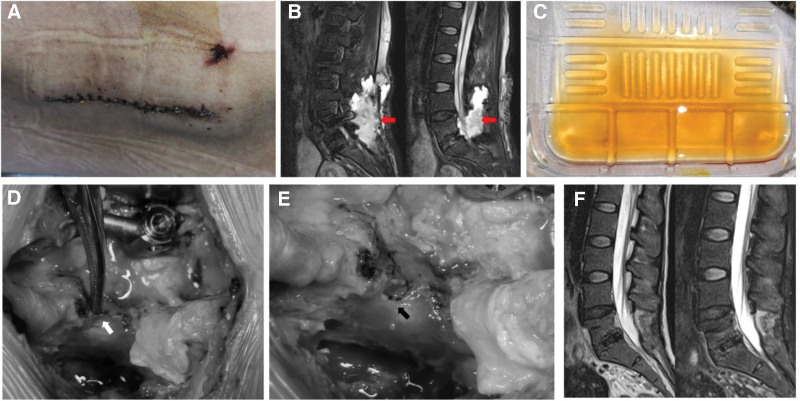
Clinical change and management of the case. (A) surgical site swelling; (B) lumbar MRI revealed significant cerebrospinal fluid (CSF) leakage; (C) drainage yielded pale-yellow transparent fluid, laboratory analysis consistent with CSF; and (D, E) intraoperative manifestation during the reoperation. (F) MRI images at follow-up. Red arrow: CSF leak in the MRI images. White arrow: the site of dural tear. Black arrow: the site of repair for dural tear. CSF = cerebrospinal fluid, MRI = magnetic resonance imaging.
